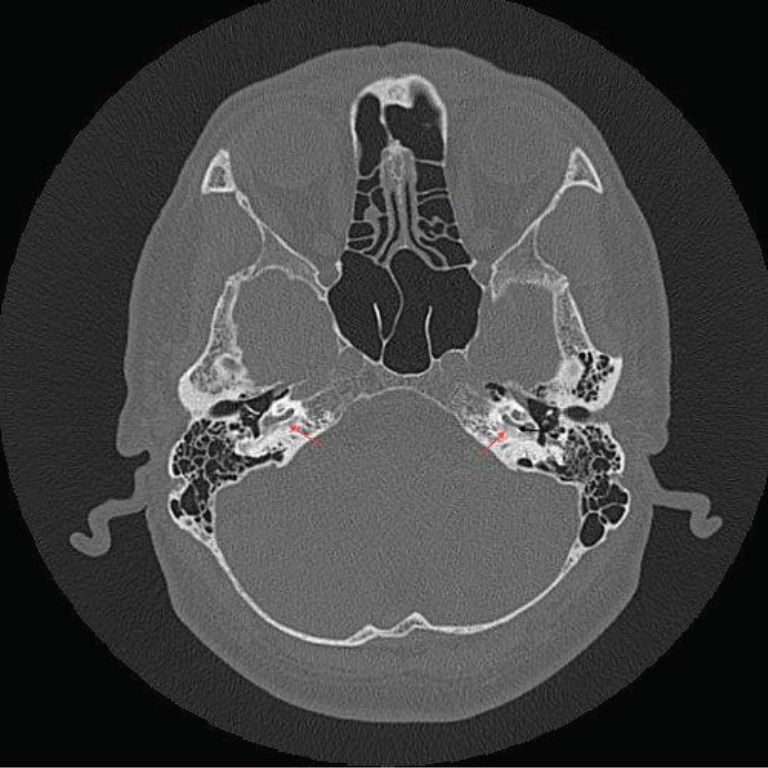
CT scan (axial plane) of patient (S8) shows bilateral retrofenestral otosclerosis (red arrows: bilateral “double ring” ; black arrow: left sided “narrowed cochlear lumen”).
